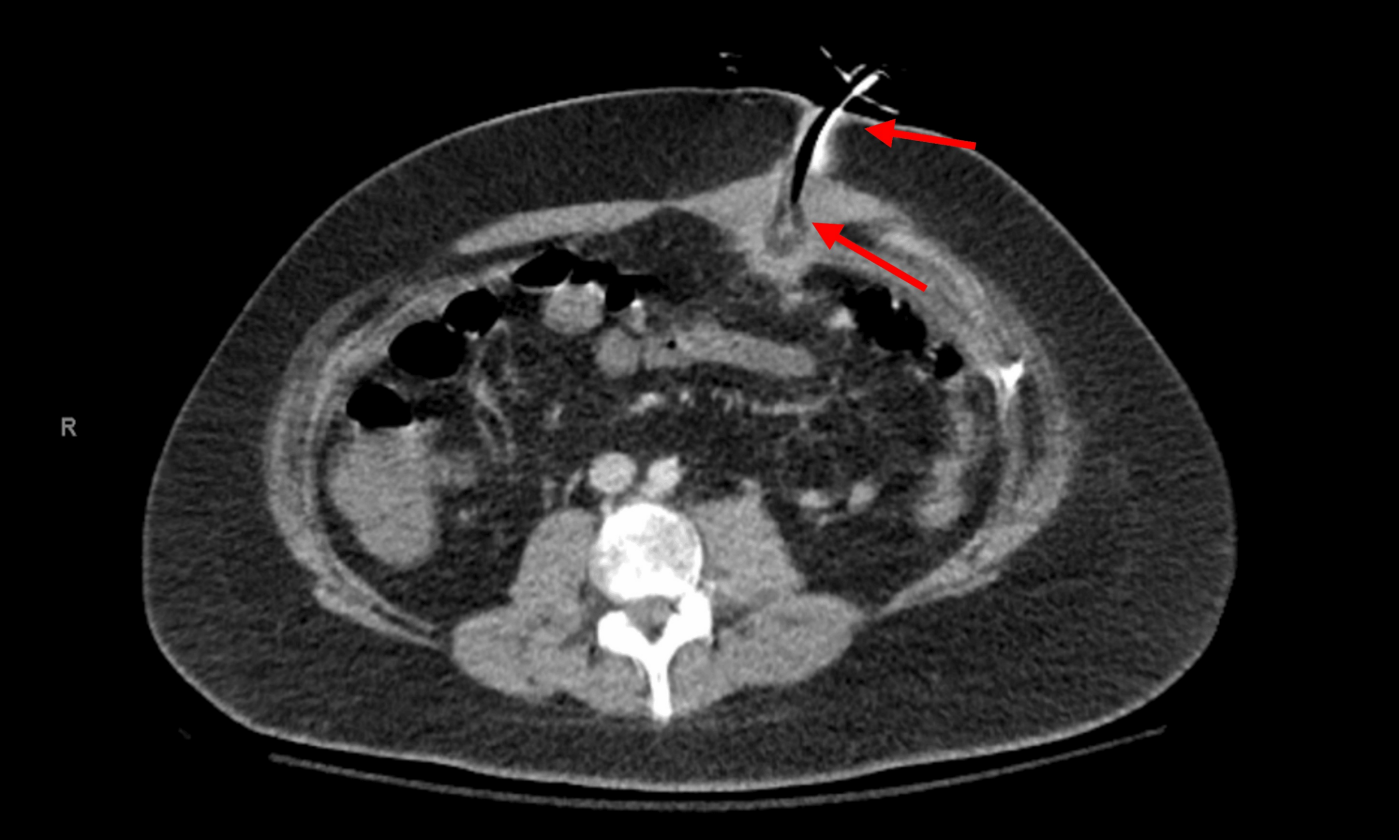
CT scan showing increased density around the percutaneous endoscopic gastrostomy (PEG) tube, with the balloon possibly located outside the gastric lumen.
